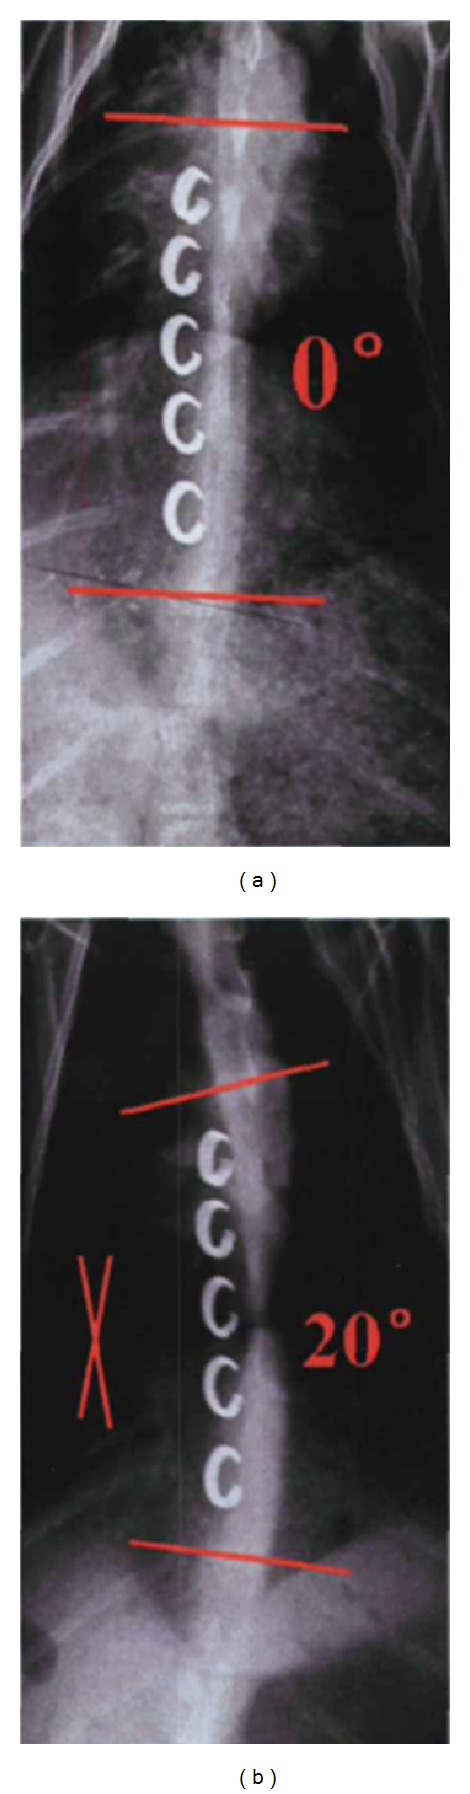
X-ray of the postoperative stability test. (a) After 2 months; (b) after 4 months.
